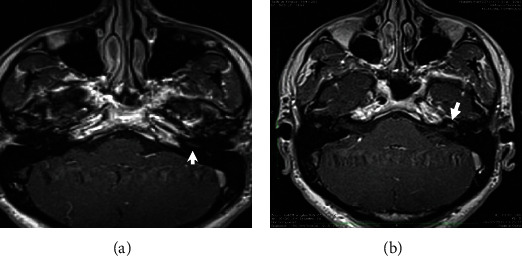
Magnetic resonance imaging of the temporal bone: axial postcontrast T1-weighted slices showing no enhancement after gadolinium injection in the basal (a) and the second (b) turns of the left cochlea.
